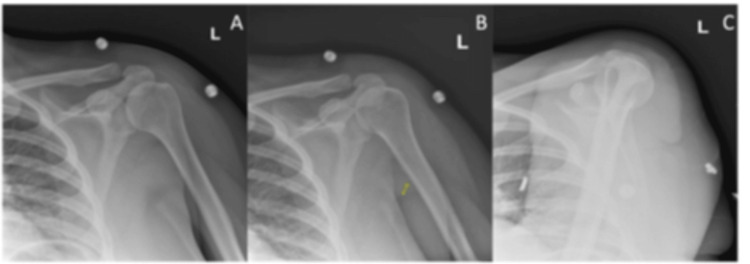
X-ray of the left shoulder showed a normal acromioclavicular joint and no acute osseous abnormalities, lesions, or arthritic changes. (A) Left shoulder external view. (B) Left shoulder internal view. (C) Left shoulder axial view.
